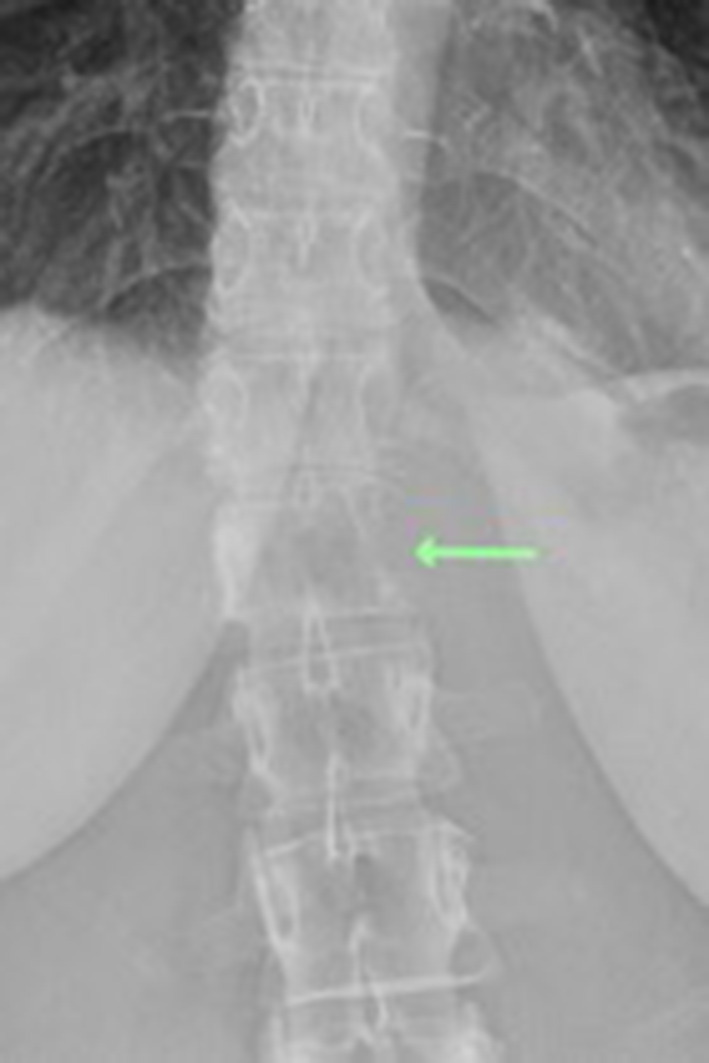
Plain radiograph demonstrating bony irregularity along the left border of T12 vertebral body and to a lesser extent the T11 level. Note there is mild curvature of the thoracolumbar junction convex to the left which may be secondary to pain.
